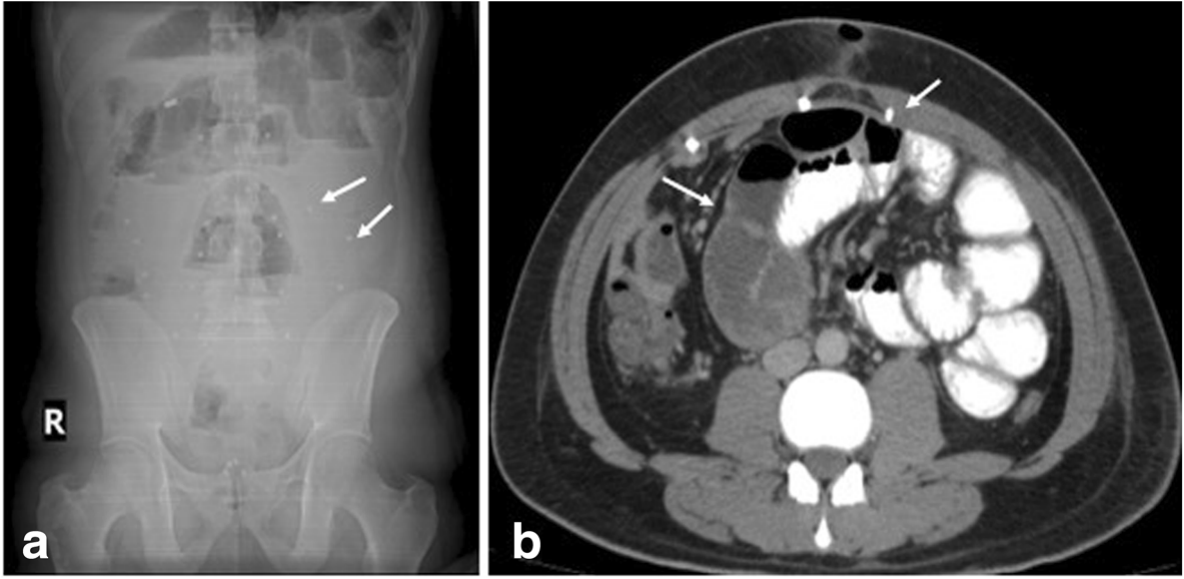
a Erect abdominal radiograph shows features of small bowel obstruction (multiple air fluid levels in dilated loops) with evidence of radiodense tackers (arrows) suggesting mesh placement. b CECT axial section of the same patient shows the radiodense tackers (short arrow) and adhesion as the cause of bowel obstruction (long arrow)
